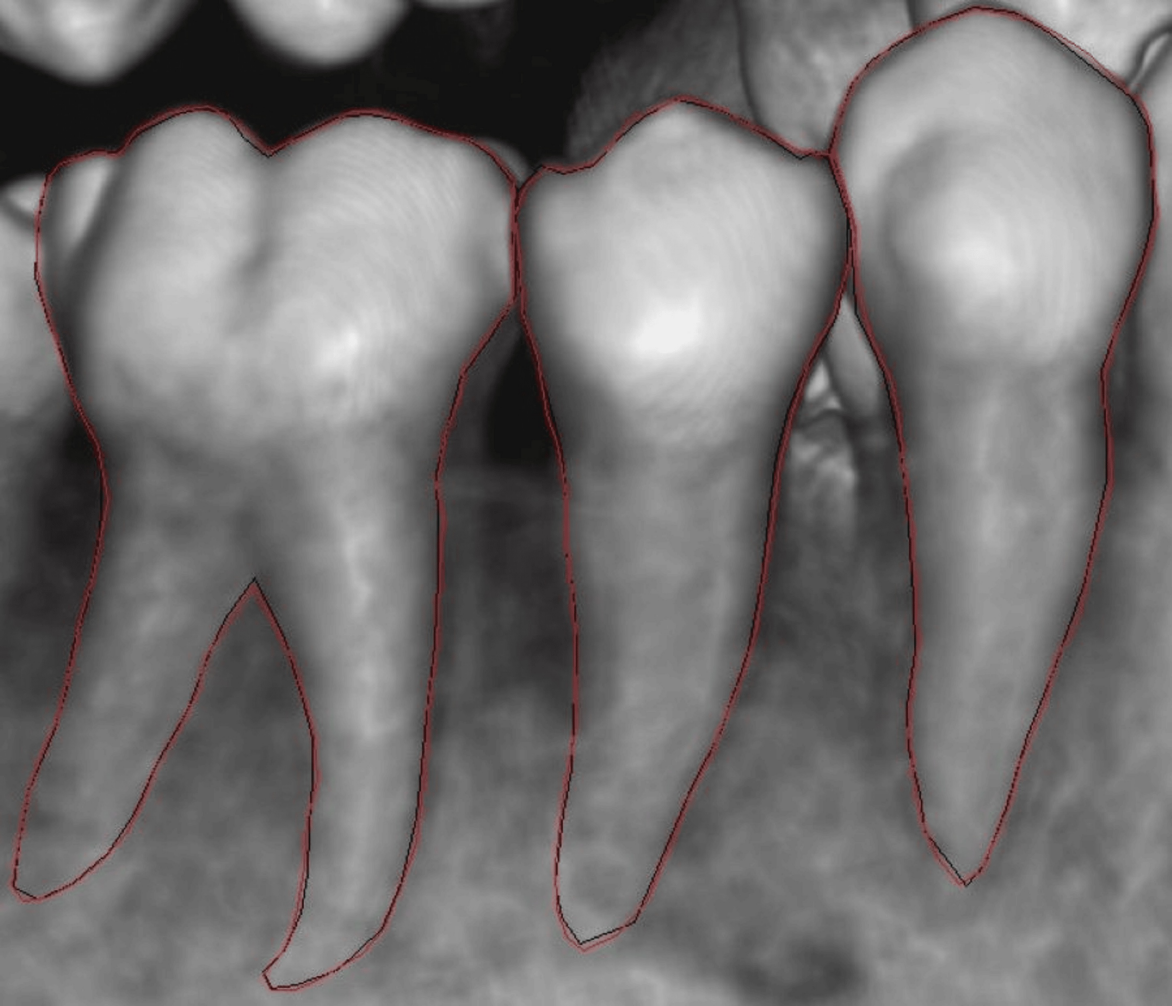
Tooth instance segmentation from a cone-beam computed tomography (CBCT) scan using You Only Look Once, version 8 (YOLOv8) software. Original image derived from the patient’s record.
